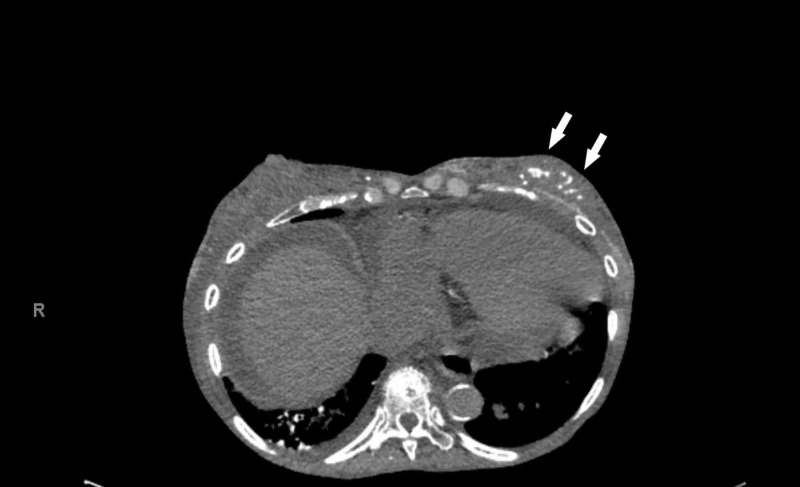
Computed tomography (CT) scan demonstrated soft tissue calcinosis (arrows)
